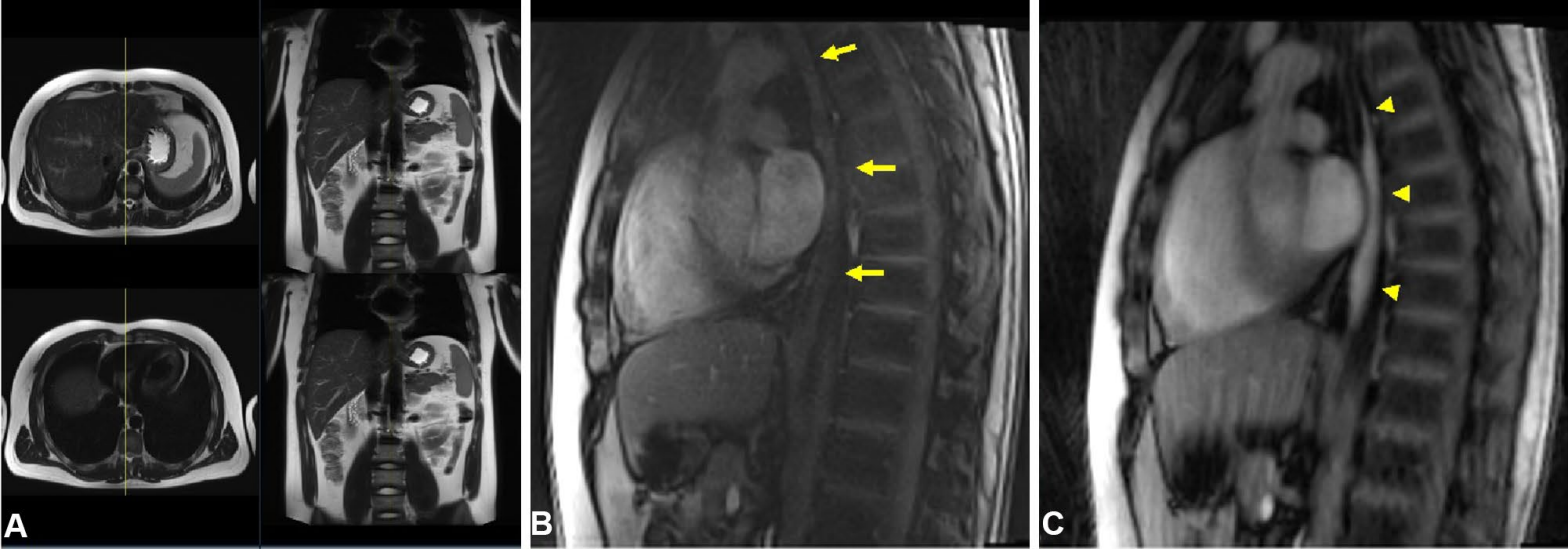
Definition of sagittal real-time MRI of the esophagus. (A) Axial and coronal HASTE planning images for sagittal real-time MRI. (B, C) Real-time MRI of the empty esophagus before bolus arrival (yellow arrows) and during bolus transit (yellow arrowheads).
